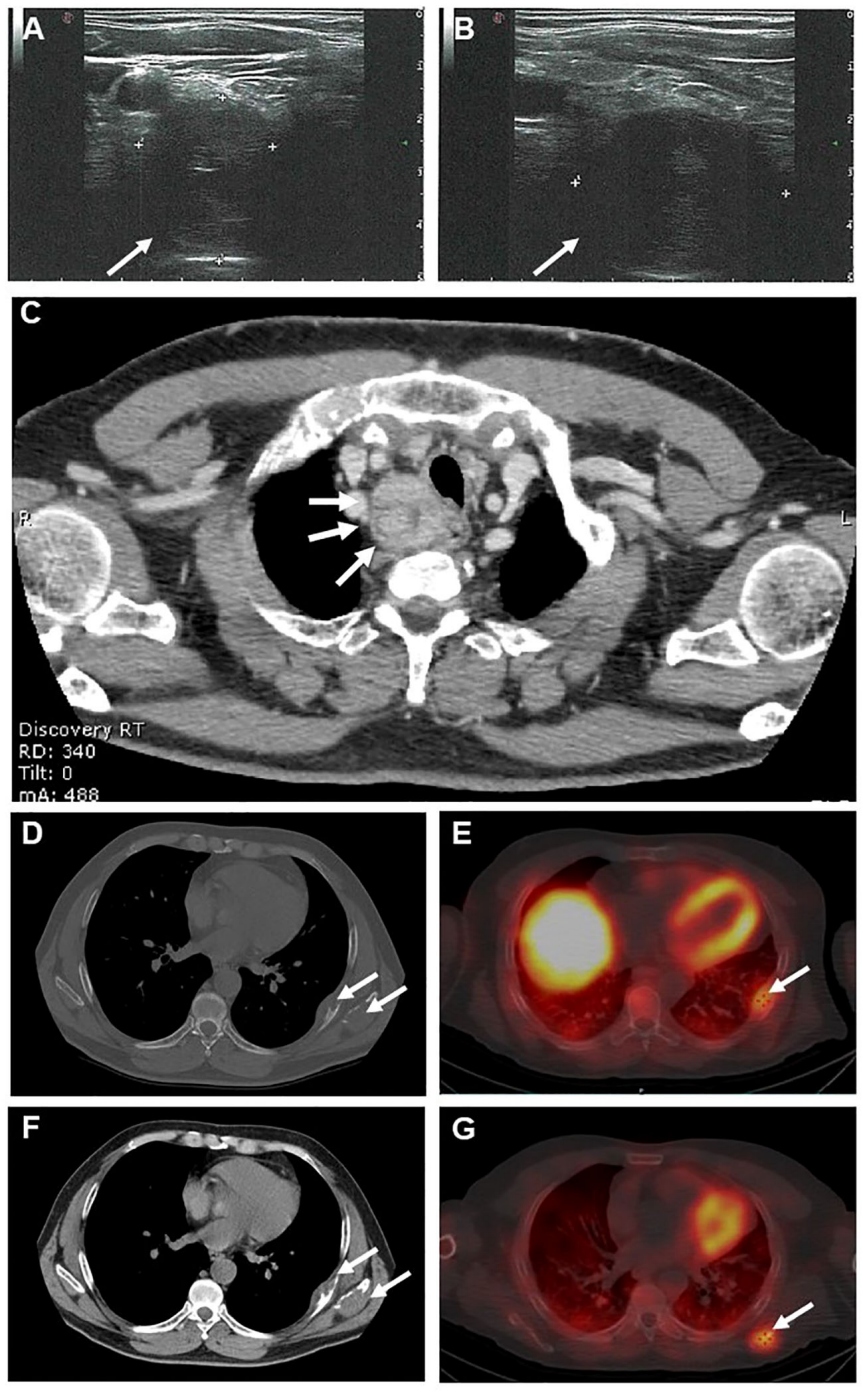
Neck ultrasound showed a 3-cm solid non-homogeneous hypoechoic nodular lesion with irregular borders, posterior to the lower pole of the right thyroid lobe consistent with an enlarged parathyroid gland (A, B). Axial contrast-enhanced neck computed tomography (CT) (C) showing a 3-cm solid heterogeneous lesion with a central area of hypodensity and irregular borders located below the right thyroid lobe (arrows). Axial section basal (D) and contrast-enhanced (F) CT scans showing two osteolytic lesions at the inferior border of the left scapula(arrow) and in the 7th rib (arrow). Axial section ^99m^Tc-sestamibi SPECT-CT scan showing uptake at the 7^th^ rib (E, arrow) and the left scapula (G, arrow).
